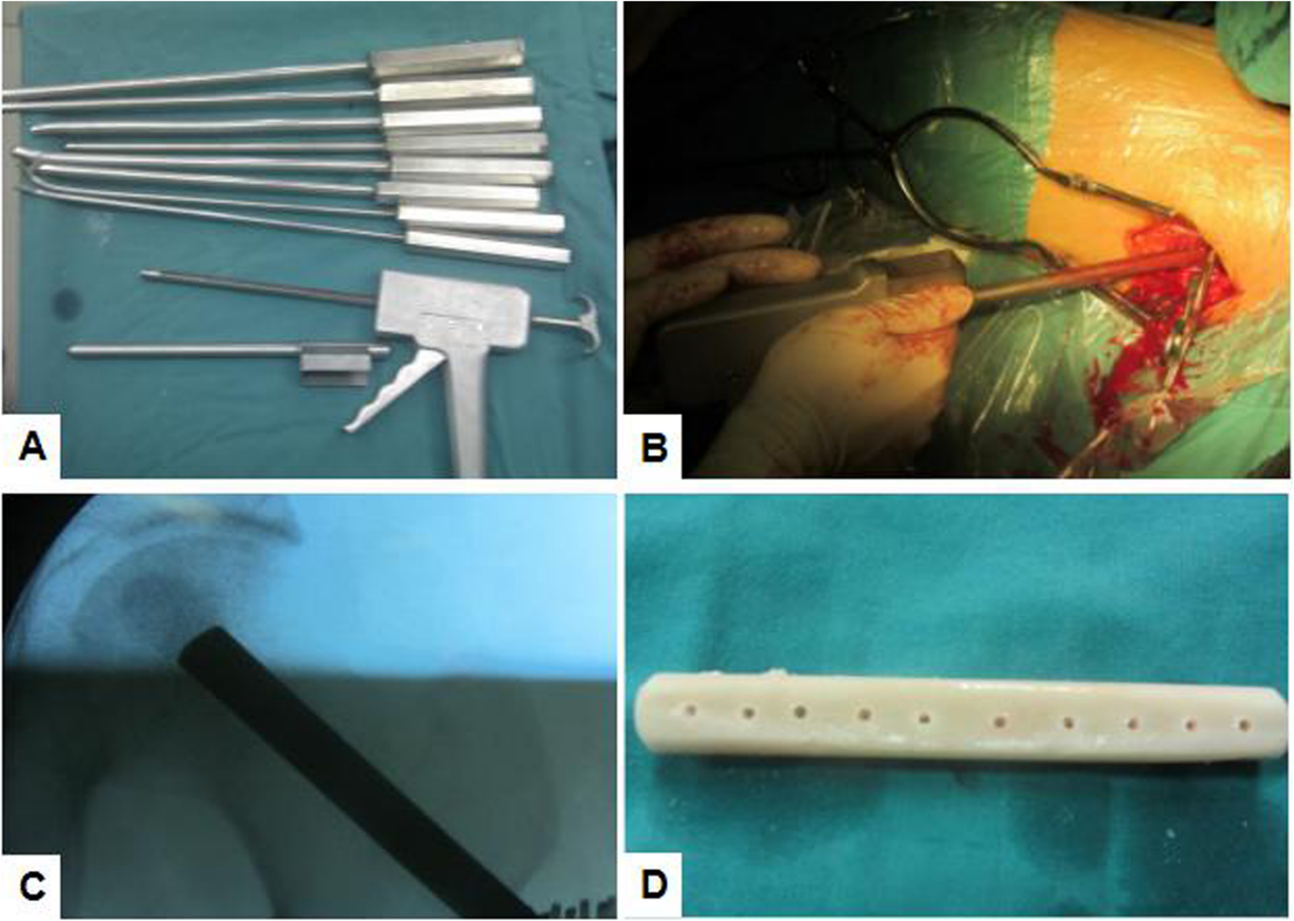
a A photograph of straight and curved impaction rods in different sizes and a bone impaction instrument. b A surgical procedure of filling cancellous bone layer by layer. c A radiograph of cancellous bone impaction grafting. d A photograph of the allogeneic fibula after drilling holes for the purpose of enhancing bone ingrowth
